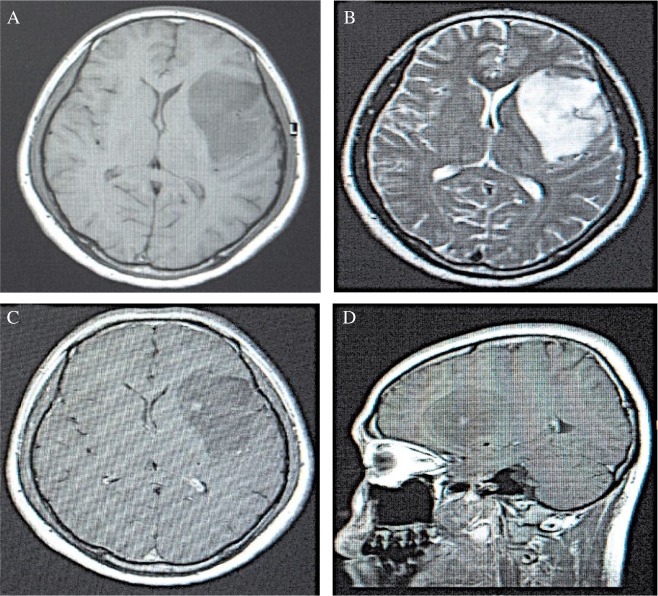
The MRI scanning result of a low grade glioma. A: A homogeneous and long T1 signal with clear border was seen in the T1WI sequence at the transverse plane in the left frontal lobe. The lateral ventricle was compressed. B: A homogeneous and long T2 signal with clear border was observed in the transverse plane T2WI sequence in the left frontal lobe, with lateral ventricle compression. C: A T1WI enhancement scanning at the transverse plane showed a slightly irregular enhancement. D: A T1WI enhancement scanning at the sagittal plane showed a slightly irregular enhancement.
